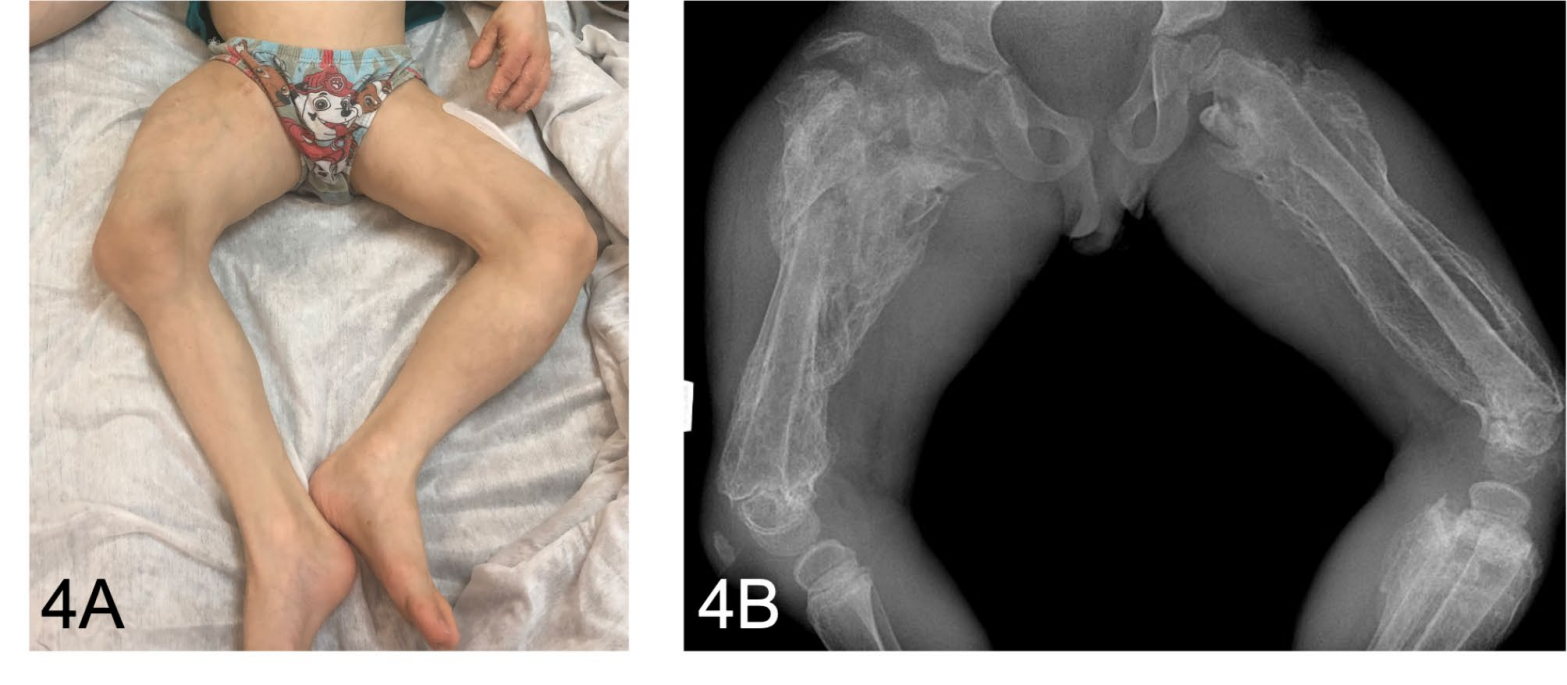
A. Photo of patient’s legs, demonstrating impossibility of full leg extension due to knee-joint contractures. B. X-ray of right and left femurs, demonstrating subperiosteal and calcified hematoma, old fractures of the right femur, osteopenia, hip dysplasia. Pseudoarthrosis with varus of the neck of the right femur with paraossal ossification
